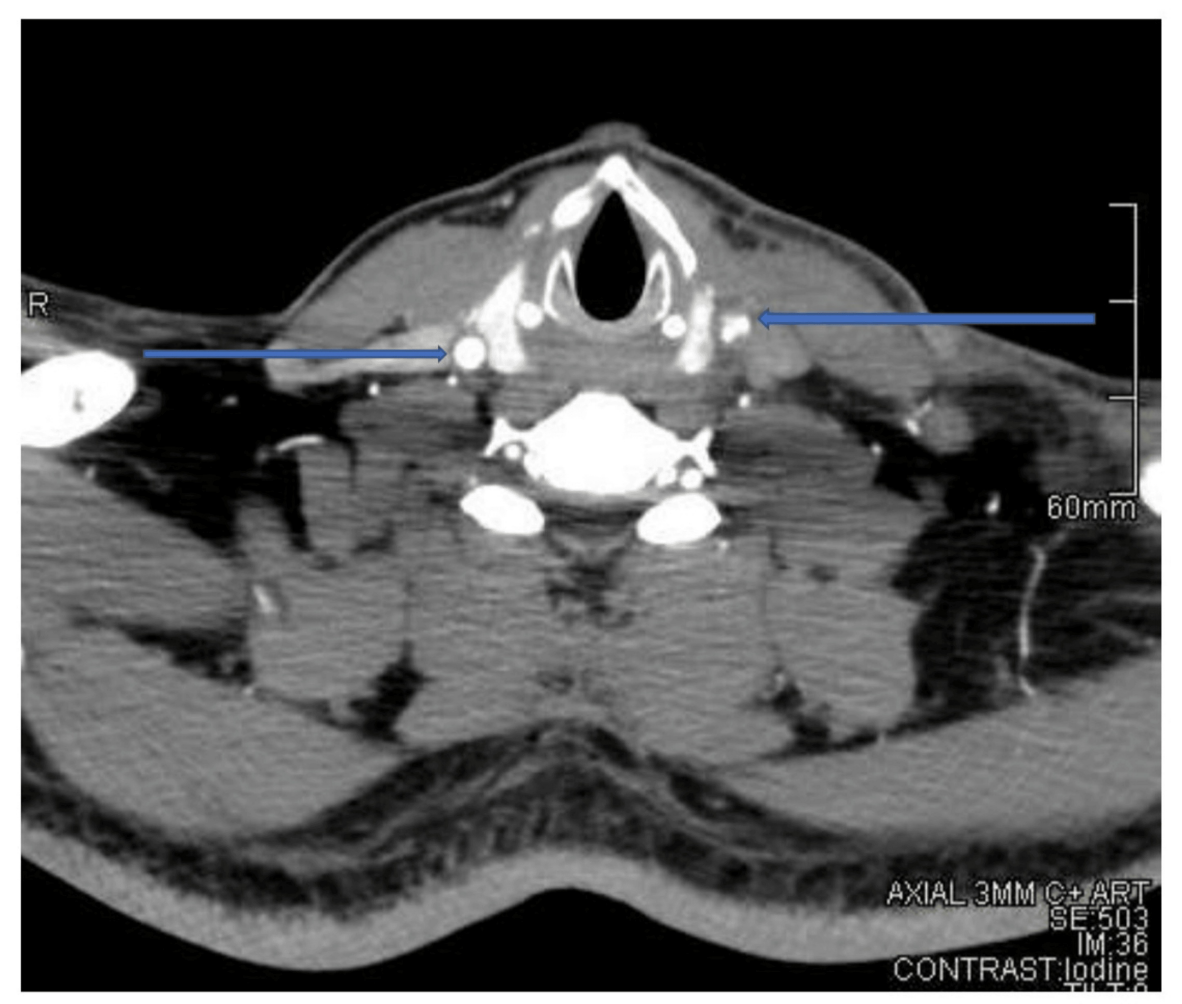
CT carotid angiogram (selected axial cut) showing abnormal left common carotid contour with associated luminal narrowing in comparison to the right
